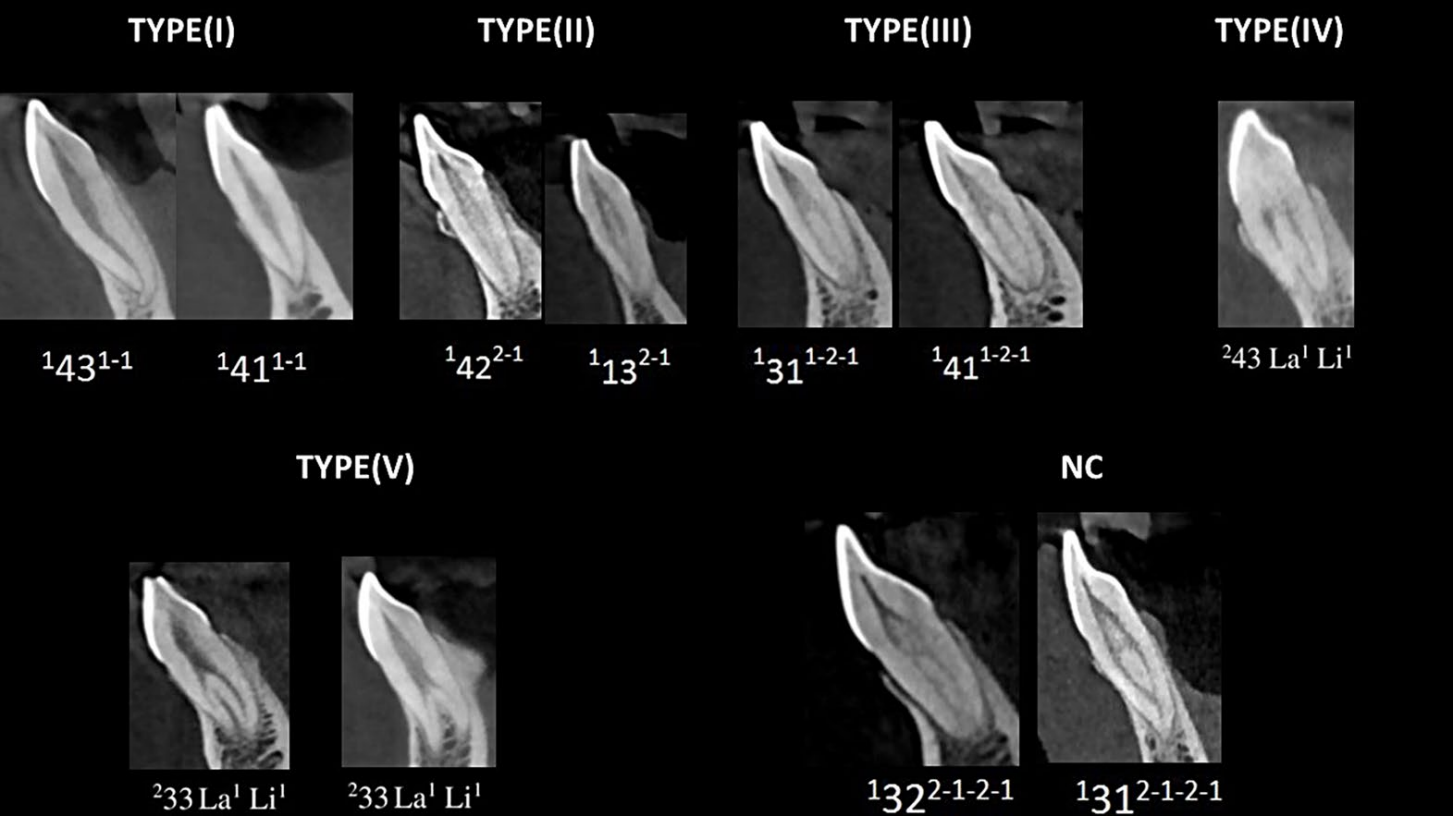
CBCT images demonstrating root canal morphological variations of mandibular anterior teeth using Vertucci and Ahmed classification
